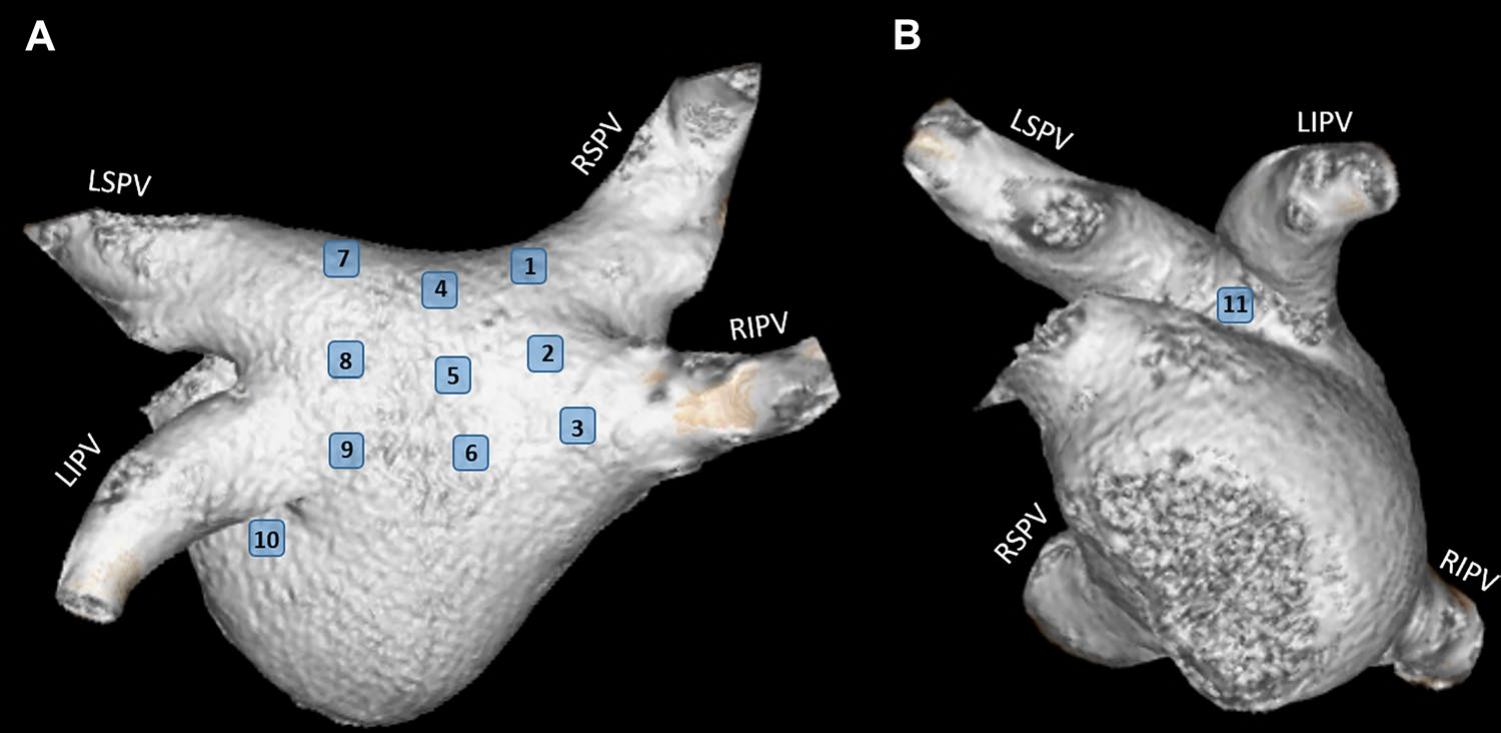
Volume rendered 3D CT images demonstrate the 11 locations where the wall thickness was measured in the left atrium (modified after Hayashi et al. [24]). On A the LA is in a posterior view whereas B represents the left lateral view of the LA. The numbers show the following left atrial areas: 1: right roof. 2: right mid-posterior, 3: right infero-posterior, 4: middle part of the roof, 5: middle part of the mid-posterior, 6: middle part of the infero-posterior, 7: left of the roof, 8: left of the mid-posterior, 9: left of the infero-posterior, 10: mitral isthmus, 11: left lateral ridge. *LA* left atrium, *LIPV* left inferior pulmonary vein, *LSPV* left superior pulmonary vein, *RIPV* right inferior pulmonary vein, *RSPV* right superior pulmonary vein
